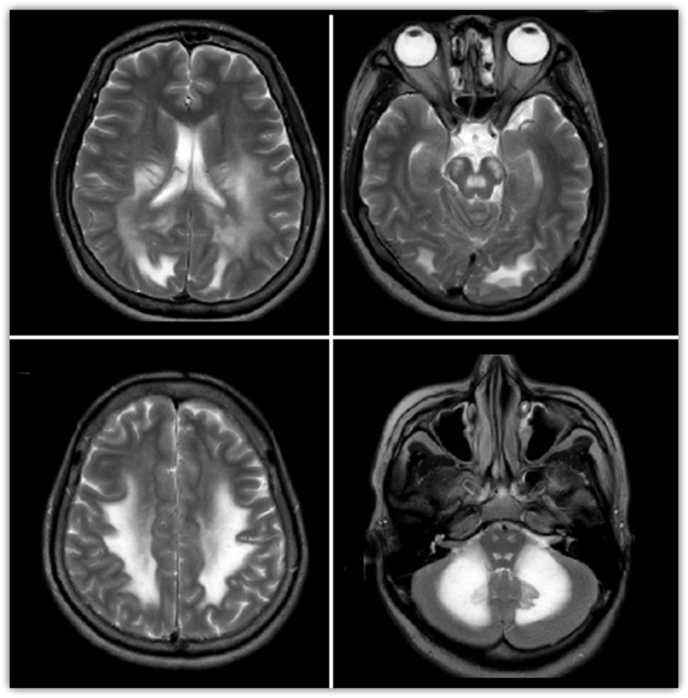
MRI of the head demonstrating extensive, symmetric T2 hyperintense signaling in the white matter of the cerebellum, brainstem, cerebral hemispheres, and some aspects of the frontal lobes.
